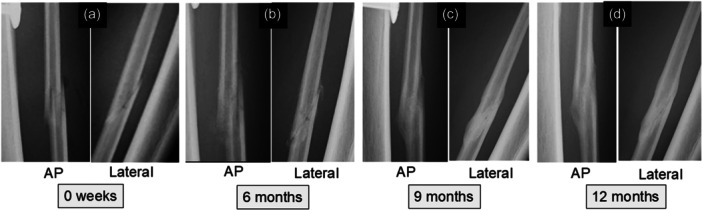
A representative patient (age, 52 years) who underwent the acute oblique osteotomy and ligation procedure. Anteroposterior (AP) and lateral radiographs of the fibular shaft were taken immediately after surgery (a), at 6 months (b), 9 months (c) and 12 months (d) postoperatively. As the radiographic union score for fibular osteotomy (RUSF) reached 12 points at 9 months postoperatively, the bone union time was determined to be 9 months.
